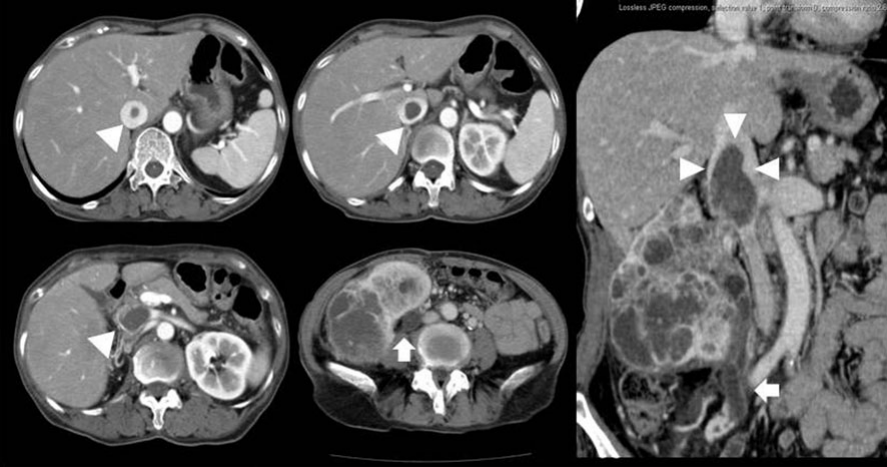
Computed tomography showed that there was a right renal cell carcinoma with a tumor thrombus extending to the site of the hepatic inferior vena cava (*arrowheads*) and that the right ureter also had a tumor thrombus (*arrows*)
